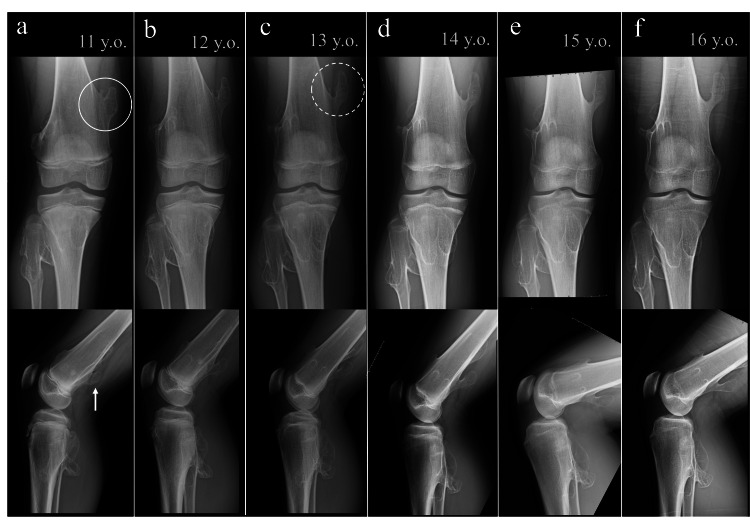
Serial radiographs of the right knee from ages 11 to 16 (a) Age 11: A sessile osteochondroma is visible on the medial aspect of the distal femur (circle), and a pedunculated osteochondroma is seen posteriorly (arrow). (b) Age 12: Both lesions gradually increased in size. (c) Age 13: The medial lesion transitioned to a pedunculated morphology (dotted circle). (d) Age 14: Both lesions remained stable. (e) Age 15: Both lesions remained stable. (f) Age 16: Both lesions persisted, and complete physeal closure was confirmed.
